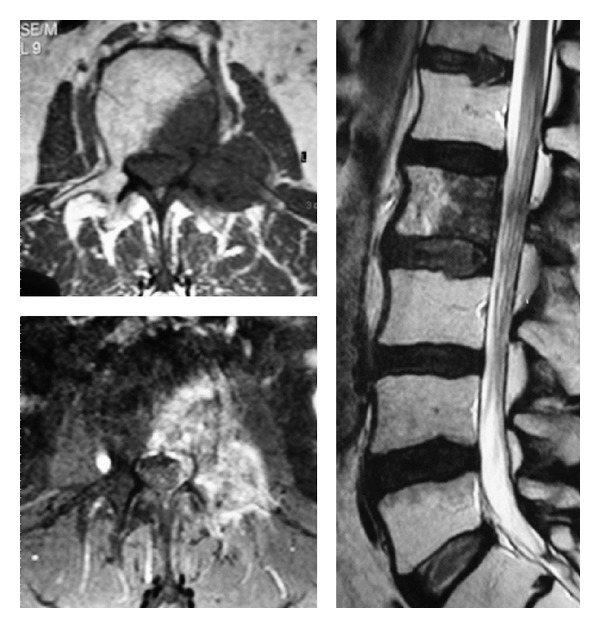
Preoperative MRI images showing an enhancing mass occupying the left hemivertebra, pedicle, and hemilamina of L2.
